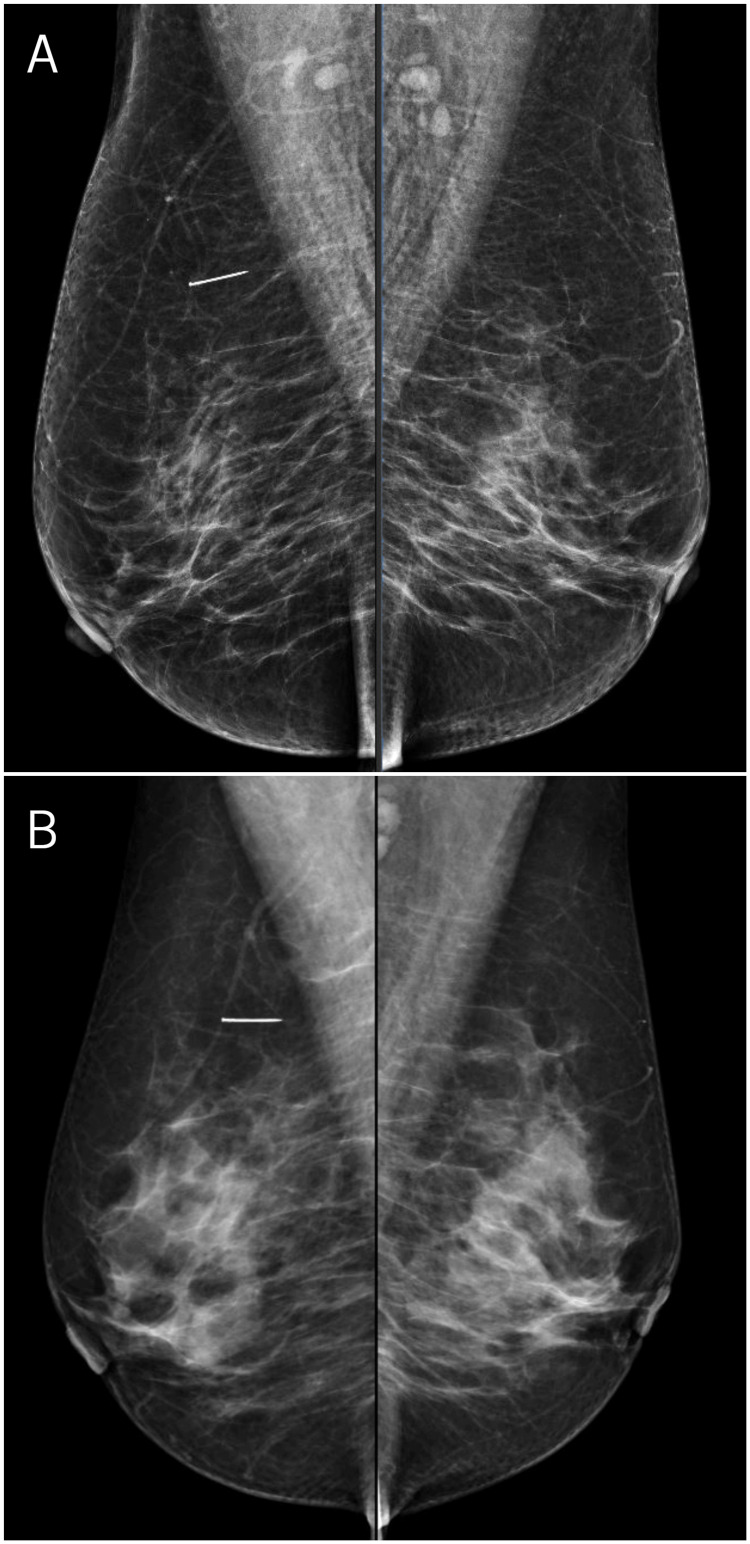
Mammography. (A) Mediolateral-oblique radiographic image from preoperative mammography in 2023.
